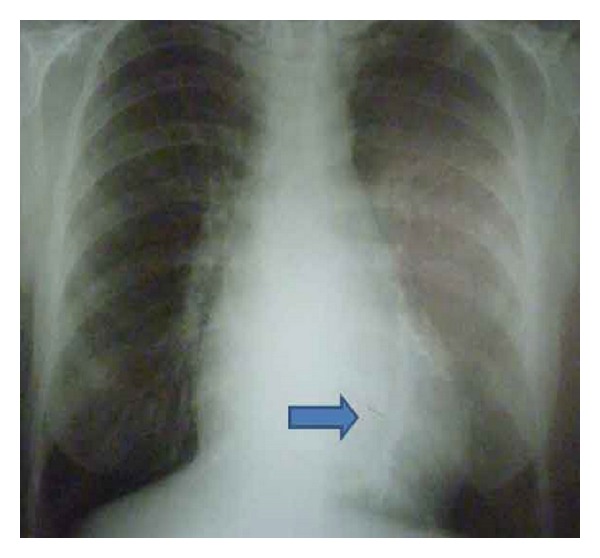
Oblique linear calcification (thick arrow) in chest X-ray.
